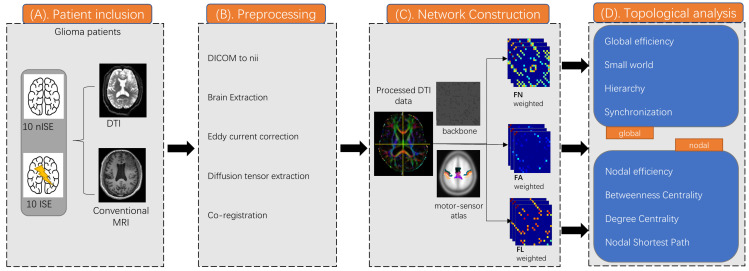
Methods and protocol. (A)Twenty patients (10 with ISE and 10 without ISE) were included and experienced DTI and conventional MRI. (B) Diffusion metrics was preprocessed by PANDA toolbox. (C) Construct a white matter connectome matrix weighted by FN, FA, and FL. (D) Global and nodal topological properties were calculated and analysed.
